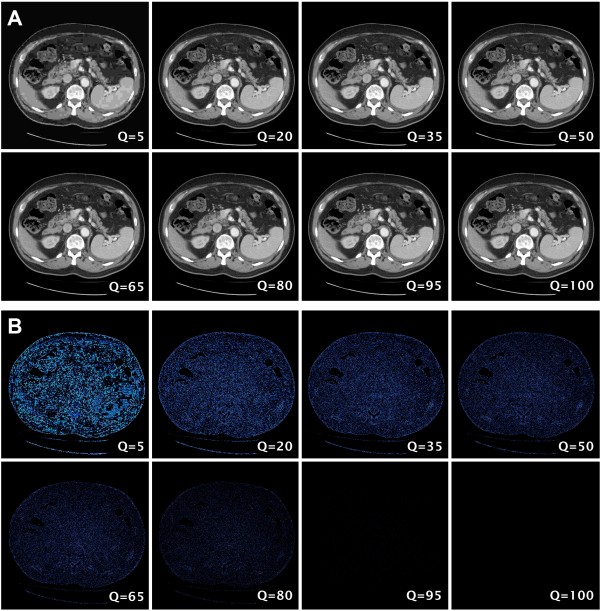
Visual demonstration of the process of image subtraction to reveal error pixels created by JPEG compression. A. A range of 8 example JPEG images from an abdominal CT scan, with compression Q values ranging from 5 to 100. B. A range of 8 images created by subtracting each of the images in (A) from the Q = 100 image. If the same window level as the original images in (A) is used, the error pixels are difficult to appreciate, so the images have been pseudocolored with a black to blue lookup table (LUT) and the contrast has been increased to better show the error pixels.
